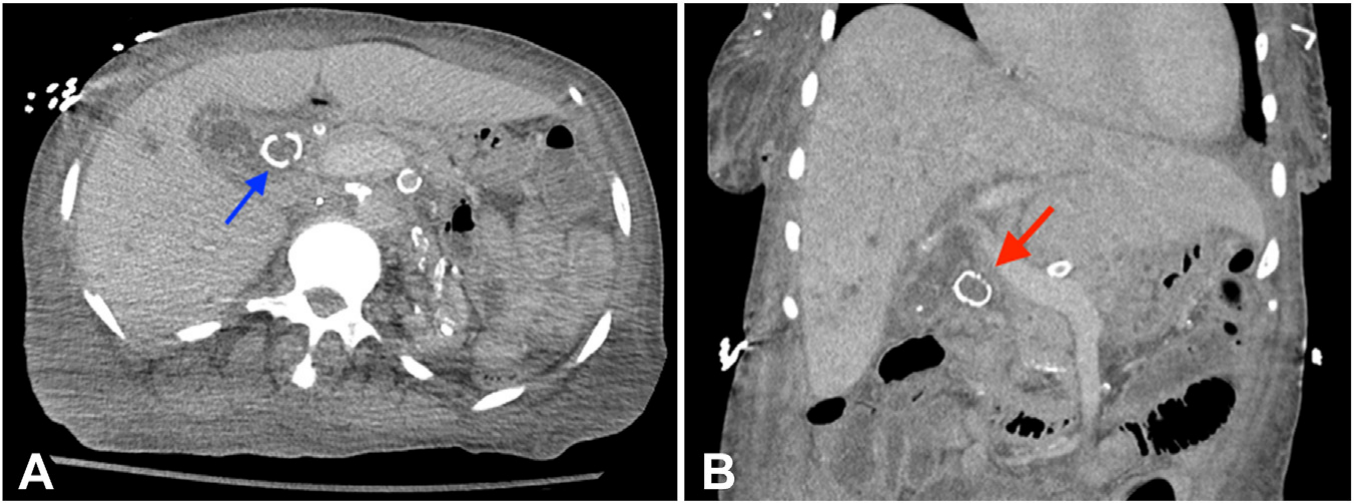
A, Abdominal CT scan (*axial*) showing a large, calcified common bile duct (CBD) stone (*blue arrow*). B, Abdominal CT scan (*coronal*) showing a large calcified CBD stone (*red arrow*).
